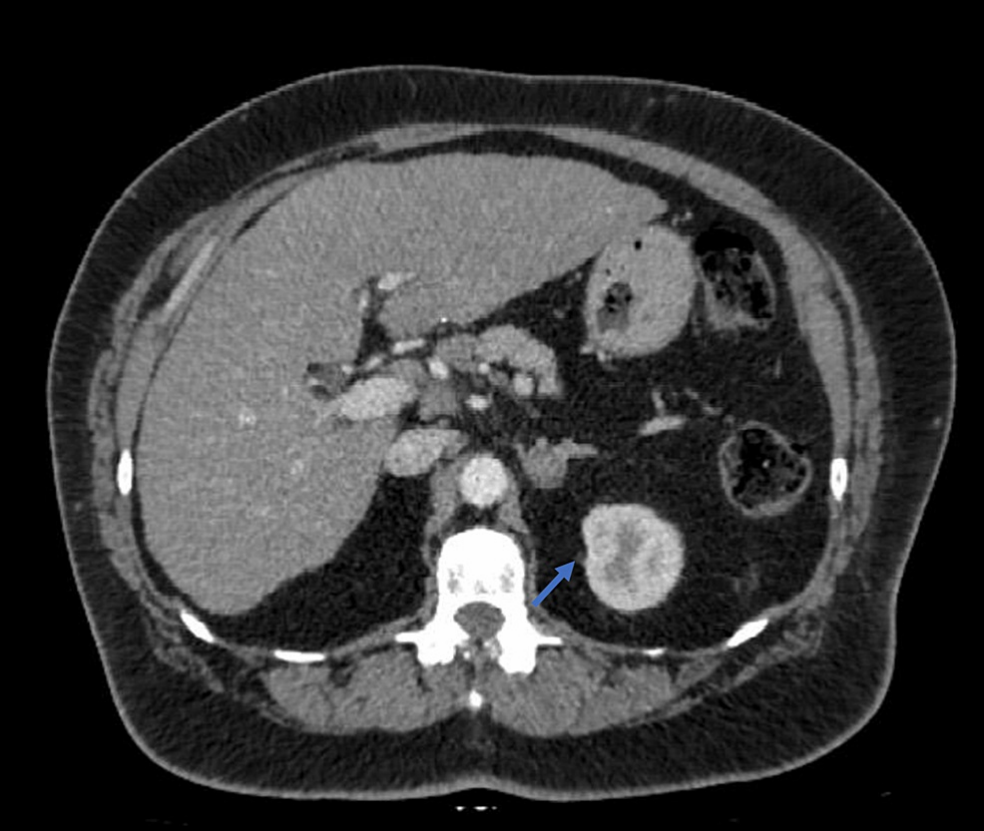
A CT scan of the abdomen with contrast shows an 18-mm nodule on the left adrenal gland, with a contrast enhancement showing a value of 56 HU (arrow). HU: Hounsfield unit
